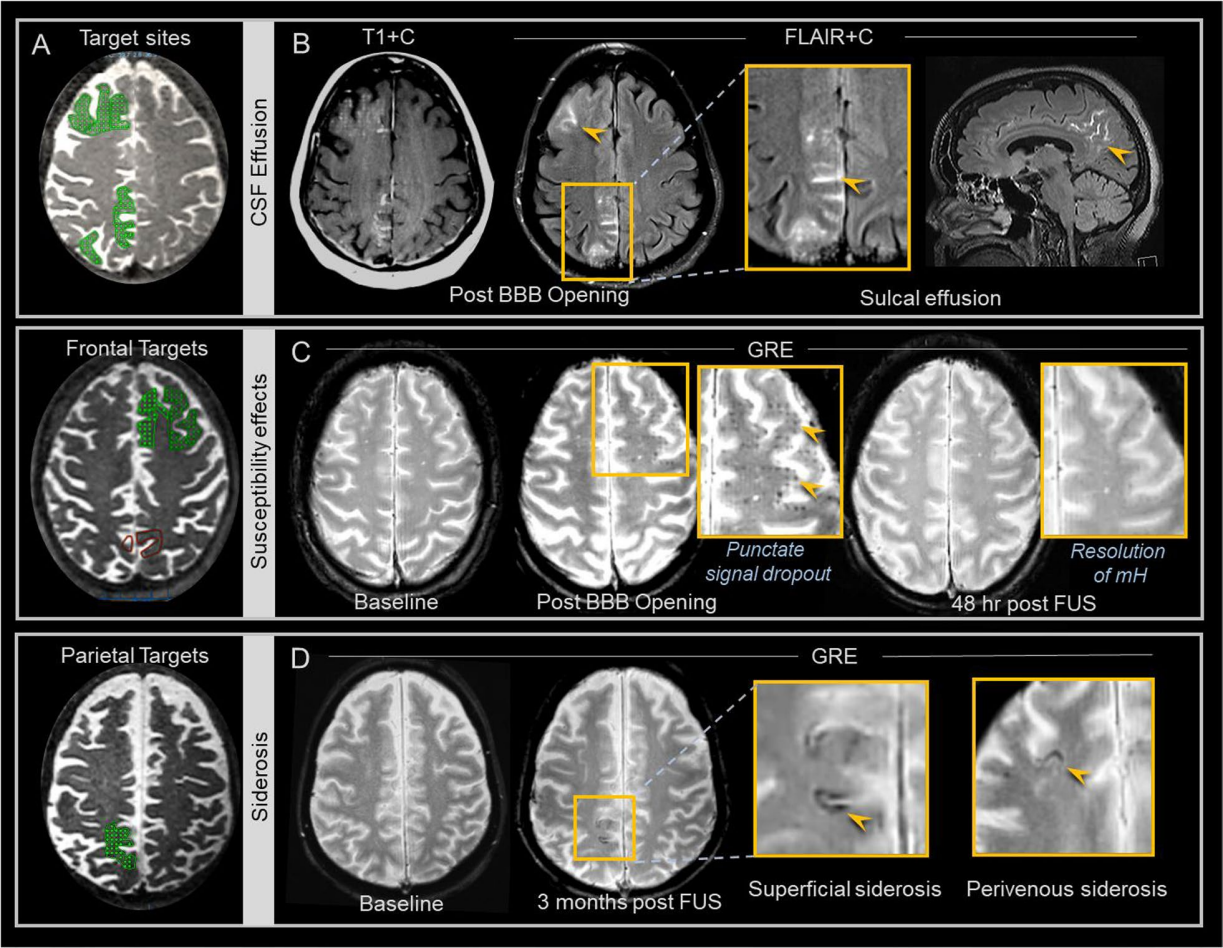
Cerebrospinal fluid effusions and susceptibility effects are observed after blood–brain barrier opening. Frontal and parietal FUS target sites are shown on T2-weighted images in three patients A. Post-contrast T1 (T1 + C) and T2-FLAIR (FLAIR + C) MRI images in a 73 year-old woman with Alzheimer’s disease (same patient shown in Fig. 2B) show CSF space hyperintensity (arrowheads), indicating CSF effusions, over frontal and parietal brain regions treated with FUS. GRE images in a 67 year-old woman with Alzheimer’s disease C show development of punctate foci of signal dropout at targeted frontal brain region (arrowheads) compared to baseline, with resolution of these signal changes by 48 h post FUS. The GRE signal changes did not always completely resolve, however were not associated with any clinical adverse effects in any subject. GRE images in a 54 year-old woman with Alzheimer’s disease D show development of superficial siderosis (arrowhead) along the surface of the treated parietal precuneus. Perivenous siderosis (arrowhead) is also shown following FUS in a 57 year-old male trial participant
